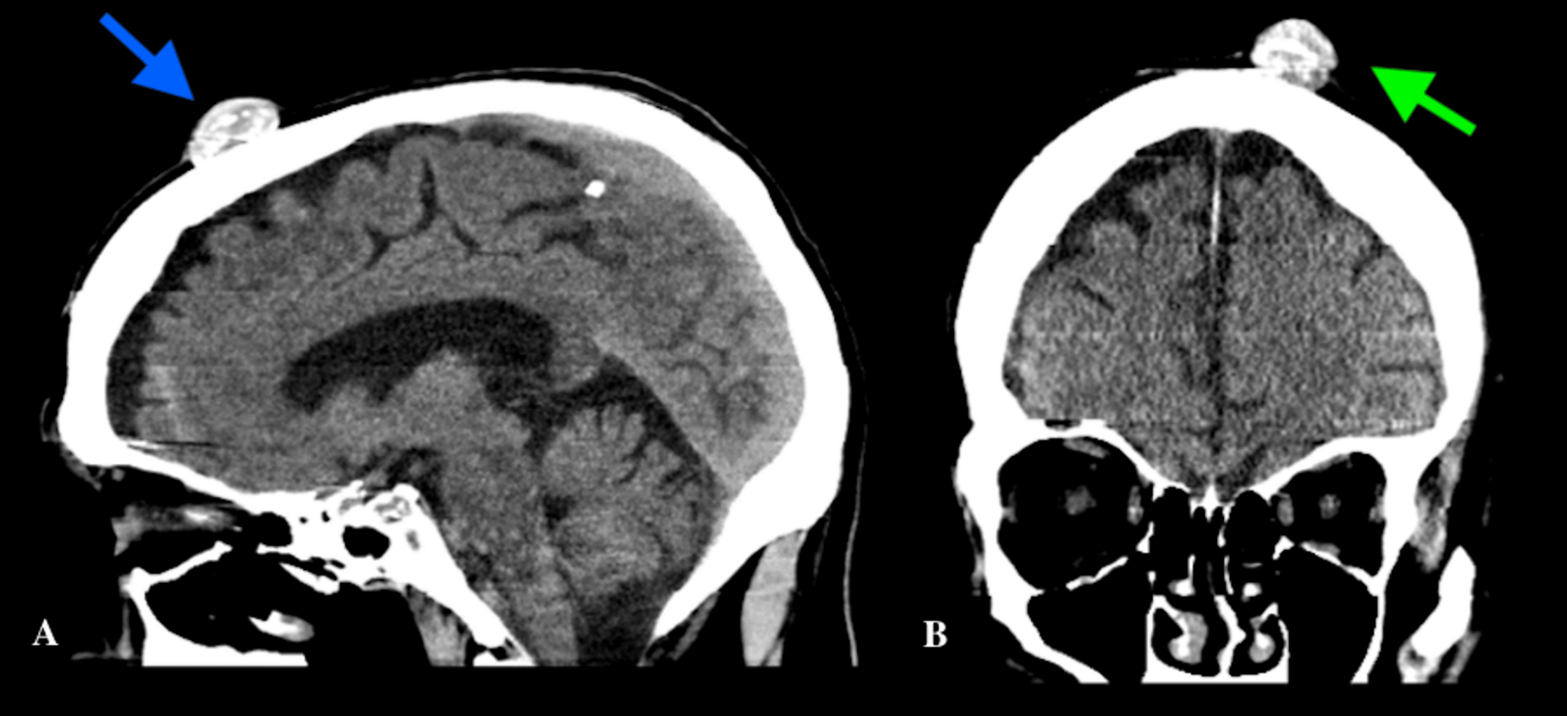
CT head of trichilemmal cyst (a) Sagittal CT view depicting mass lesion (blue arrow) and (b) coronal CT view displaying the same tissue lesion (green arrow)
